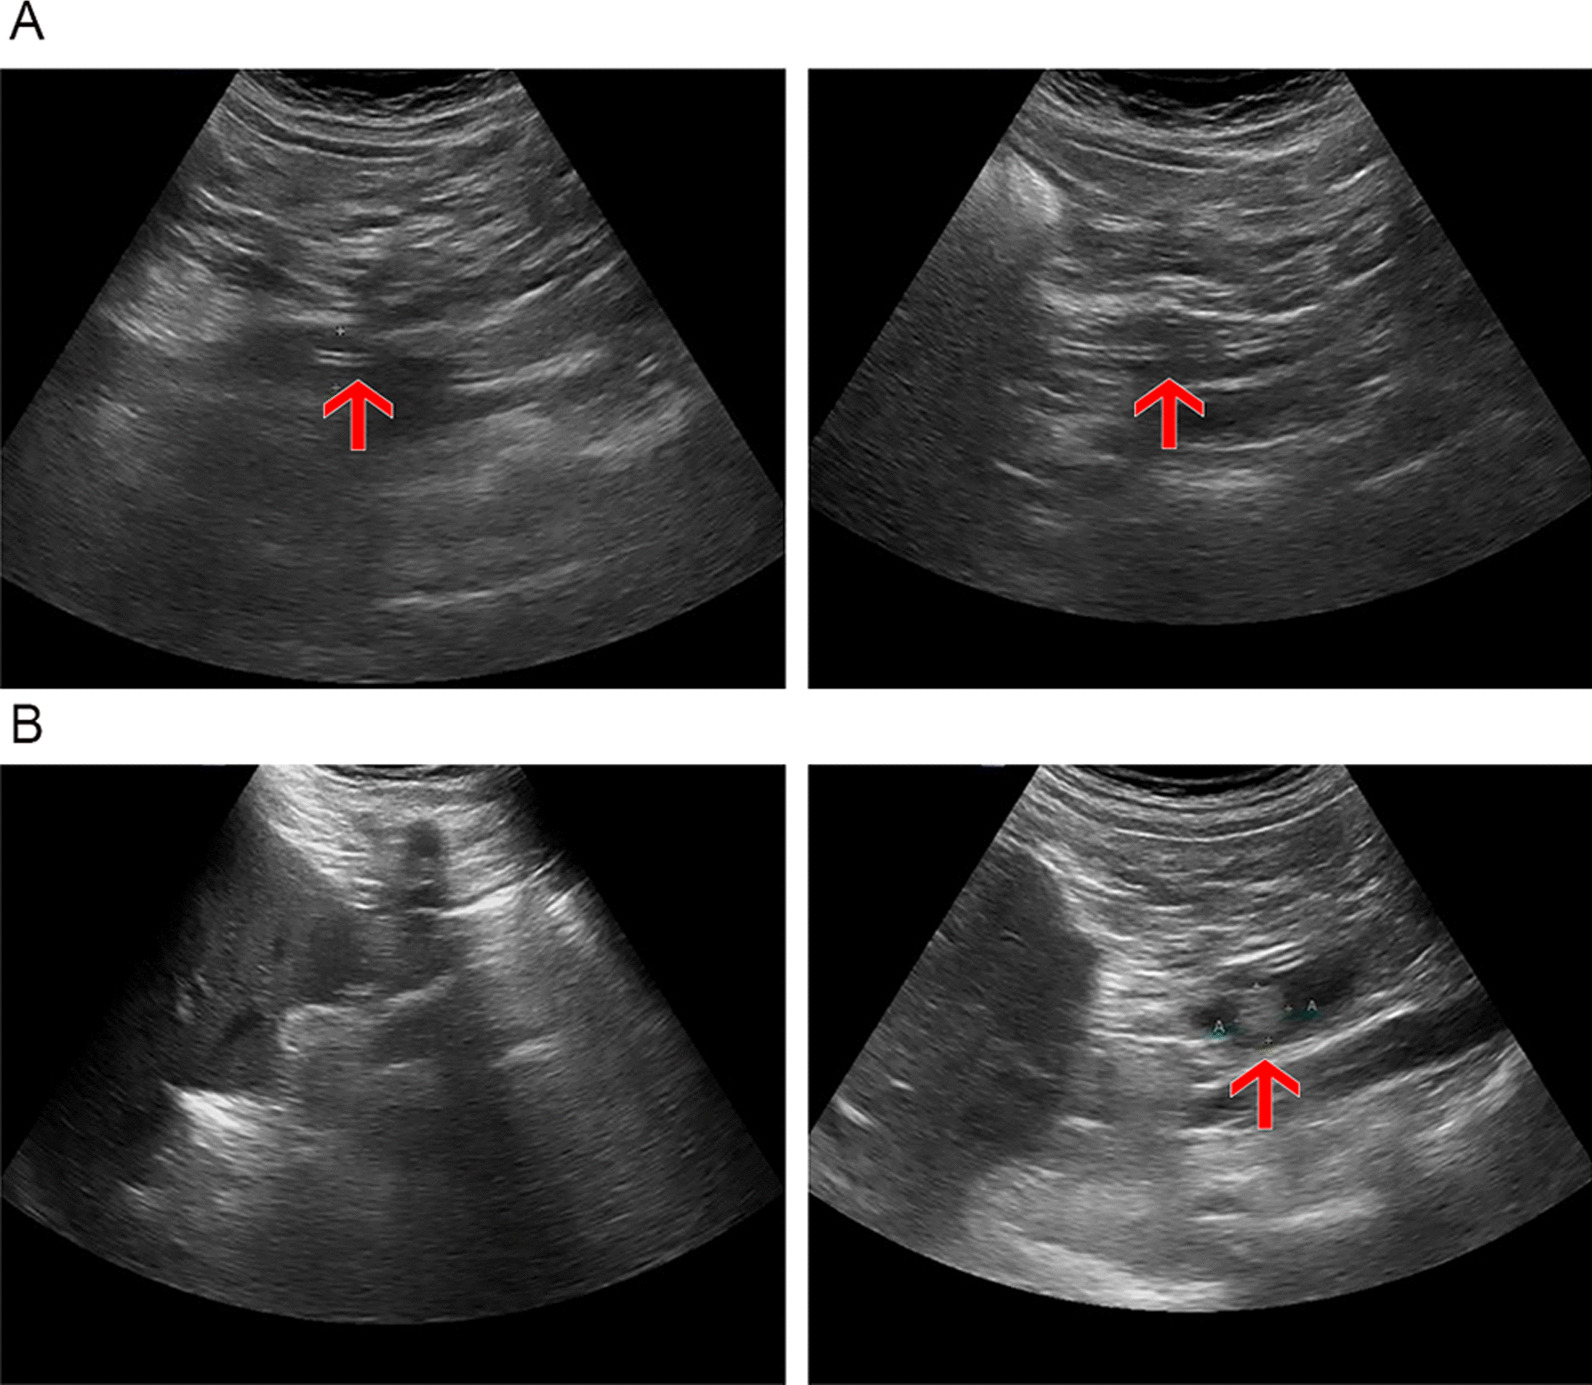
Comparison between routine ultrasound and ENBD saline injection ultrasound in a 78-year-old women. A. The imaging length of CBD in ENBD saline injection ultrasound (right) is significantly longer than in routine ultrasound (left). B. Remnant stone in ENBD saline injection ultrasound found (right) that cannot be found in routine ultrasound (left)
